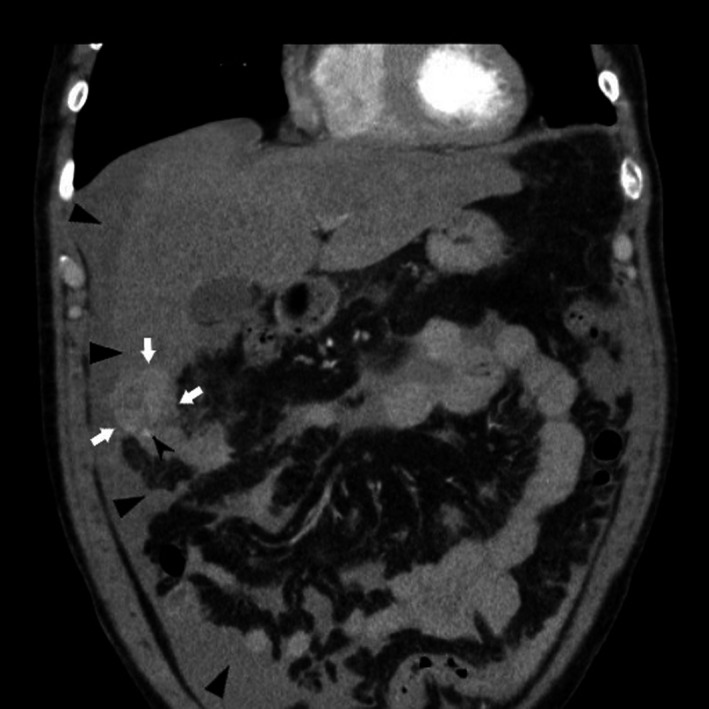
A CT scan in 2010 revealed a ruptured HCC in segment 6 of the liver (white arrows) and a contrast medium extravasation (black arrow). Free fluid (blood) was present in the abdominal cavity (black triangles). CT, computed tomography; HCC, hepatocellular carcinoma
